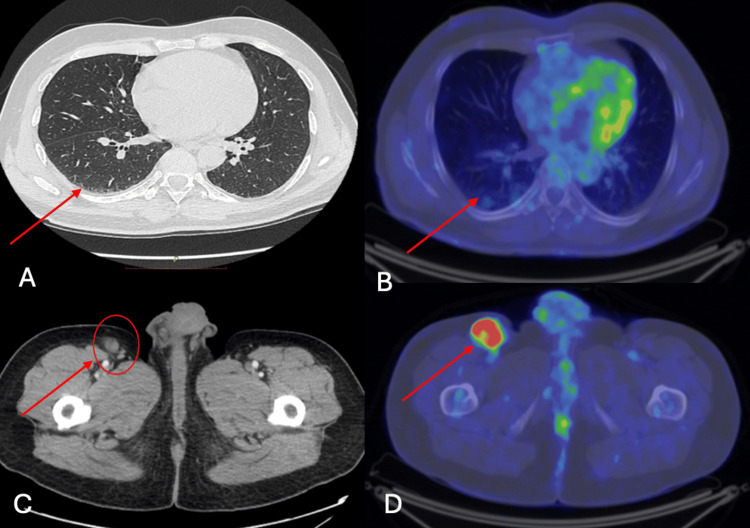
Baseline CT scan and PET-CT A: CT scan - rare lung micronodules - to be monitored. B: PET-CT - metabolically inactive bilateral lung nodules. C: CT scan - 47/33 mm right inguinal lymphadenopathy. D: PET-CT - confirmed metabolically active right inguinal lymphadenopathy.
